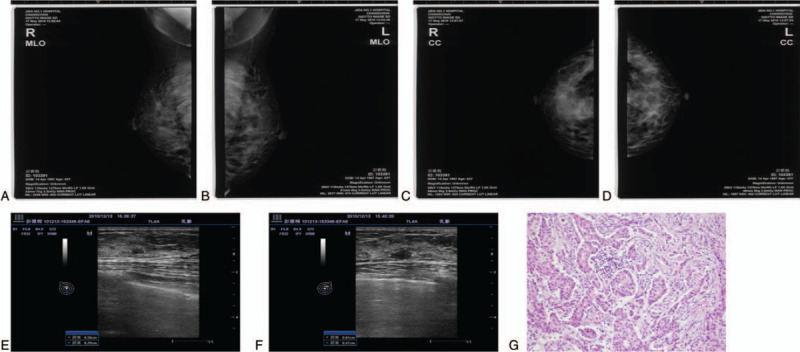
Examination results for a 36-yr-old female patient with a family history of breast cancer. (A-D) First mammography examination showing no obvious abnormal mass. (E-F) Color Doppler ultrasound images during follow-up at intervals of 3 mo showing that the tumor grew more than 30%. (G) Representative image from the pathologic examination, which confirmed the breast lesion as cancerous.
